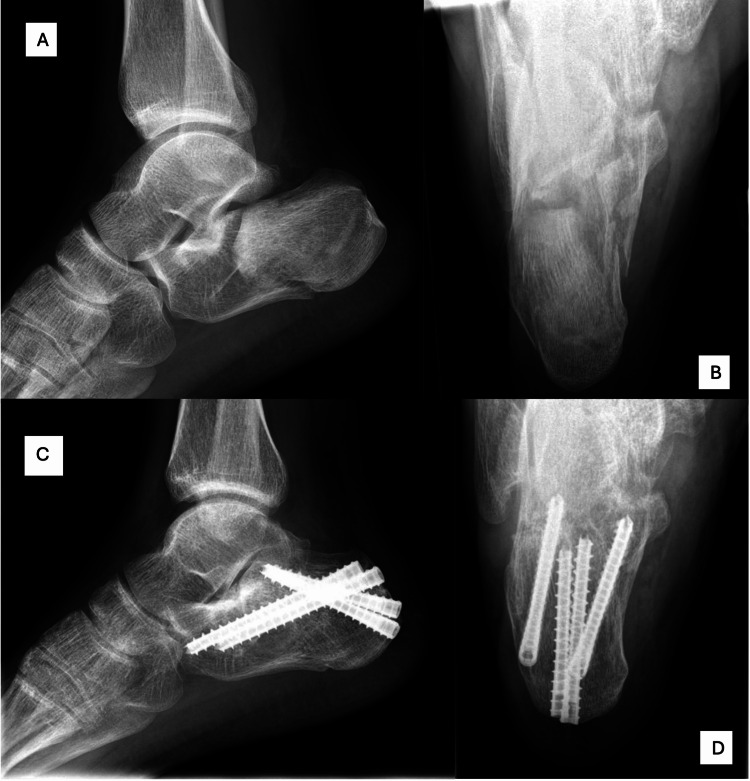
Preoperative and postoperative radiographs of the calcaneum. (A-B) Preoperative radiographs of the calcaneum and (C-D) postoperative radiographs of the calcaneum following percutaneous fixation
